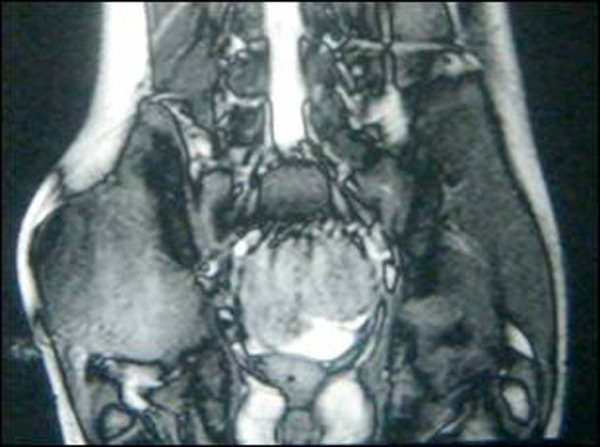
Figure 1: MRI of right gluteal region
